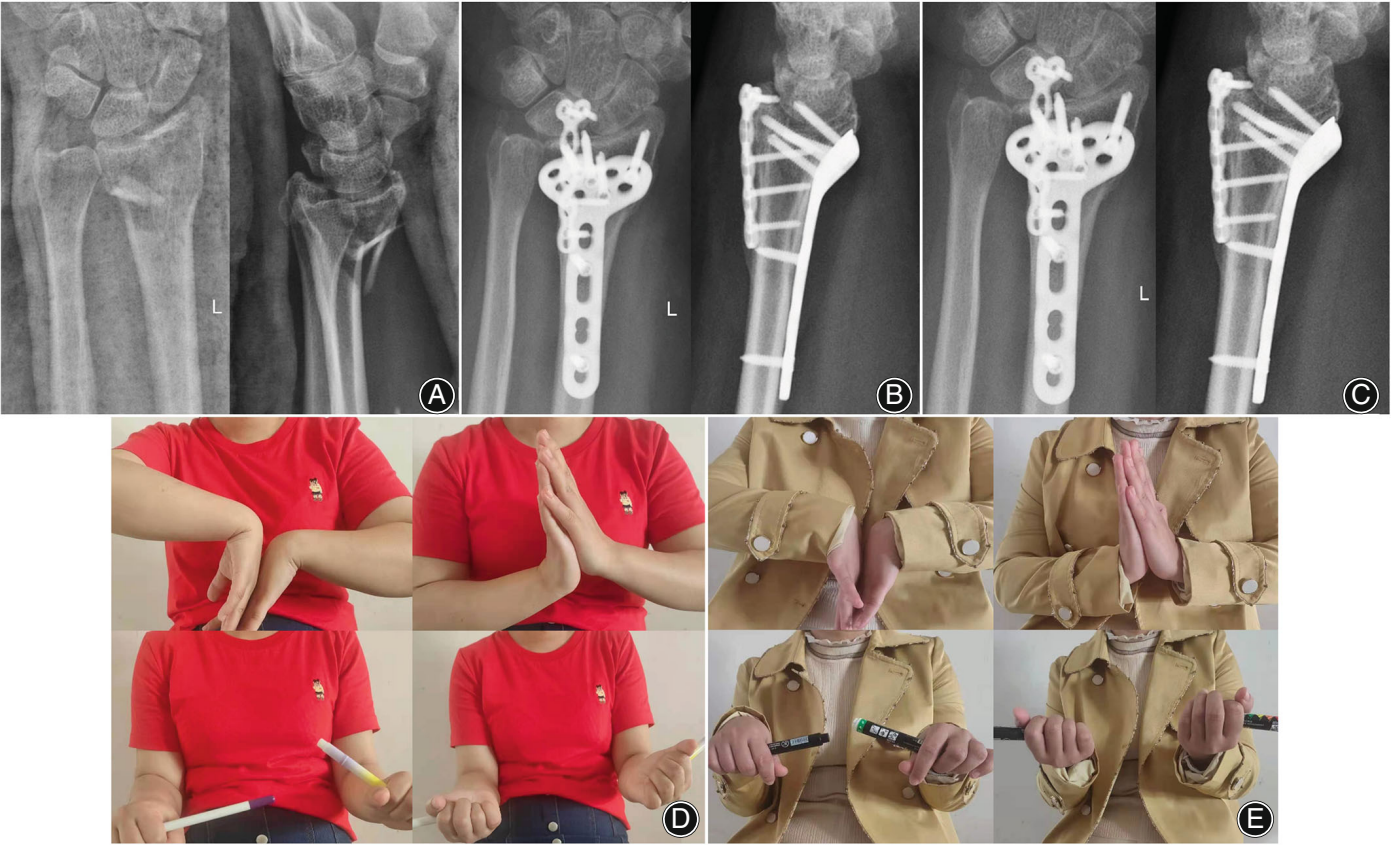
A 45‐year‐old woman suffered from malunion of distal radius fractures, with obvious fracture displacement and the loss of volar tilt. (A) Anteroposterior and lateral radiographs of the left radiocarpal joint. (B) Corrective osteotomy with volar and dorsal plate fixation restored volar tilt at 3 months postoperatively with anteroposterior and lateral radiographs shown. (C) Anteroposterior and lateral radiographs of the radius length and volar tilt within the normal range at 18 months postoperatively. (D) Wrist function was evaluated by flexion and extension of wrist and pronation and supination of the forearm at 3 and (E) 18 months postoperatively
